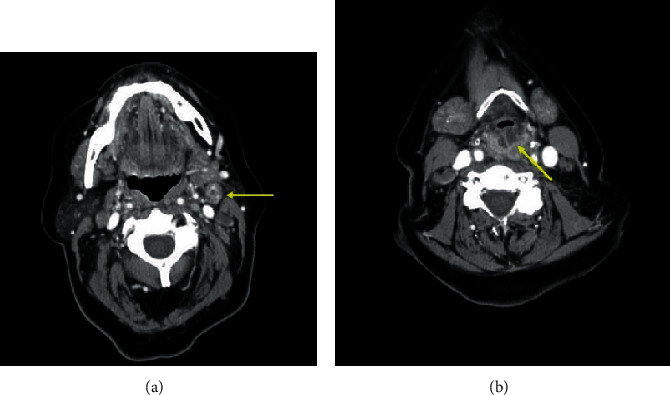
Axial nonenhanced computed tomography scan of the neck. (a) Necrotic cervical lymph nodes at level IIa and (b) submucosal edema of the supraglottis.
